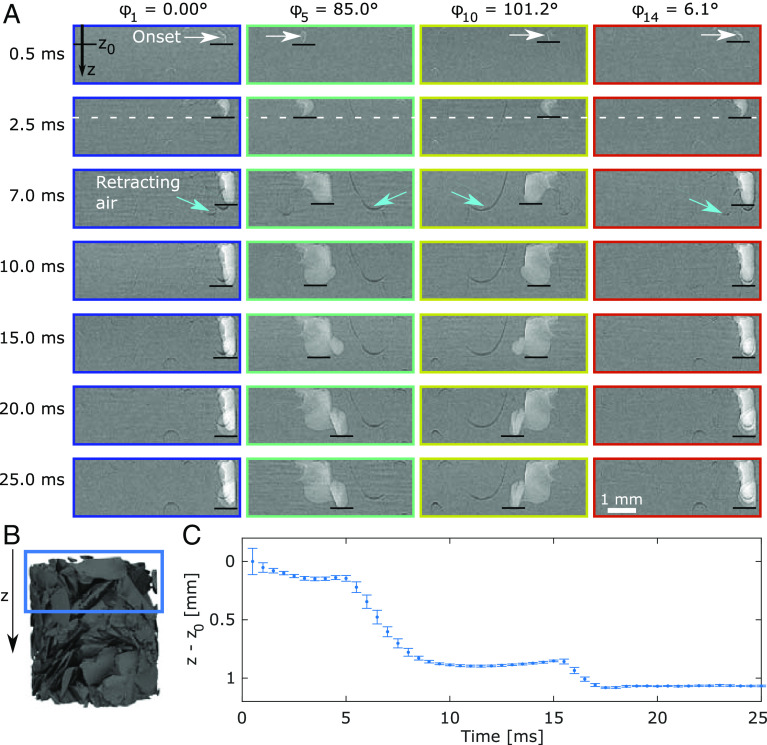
Repeatability of Haines jump H9. (*A*) Selected radiographs from four stroboscopic time series measured at projection angles $\phi_{i}$, zooming in on H9 in space and time. Corresponding radiographs obtained 50 ms prior to the onset of the jump were subtracted from each of these images to cancel the stationary structures, thereby highlighting the dynamics. Bright regions represent intruding air, with the white arrows indicating the onset of the jump in the top row. The small dark features from 7.0 ms onwards (teal arrows) are water-filled regions recently occupied with air which has retracted. The black horizontal lines indicate the farthest advanced point on the intruding air–water meniscus. (*B*) Region of interest used in (*A*). (*C*) Position of the farthest advanced point on the meniscus, averaged over the 14 projections, with the error bar signifying one SD. Larger deviations can be observed where the interfacial velocity was higher, i.e., around 7 and 16 ms.
